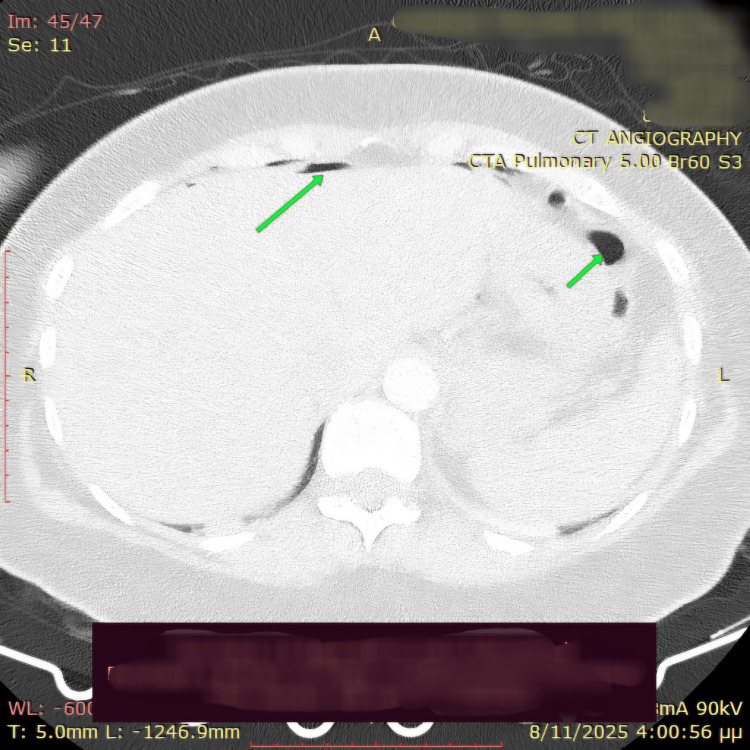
Computed tomography axial image demonstrating pneumoperitoneum, with the presence of free air in the abdominal cavity (arrows).
